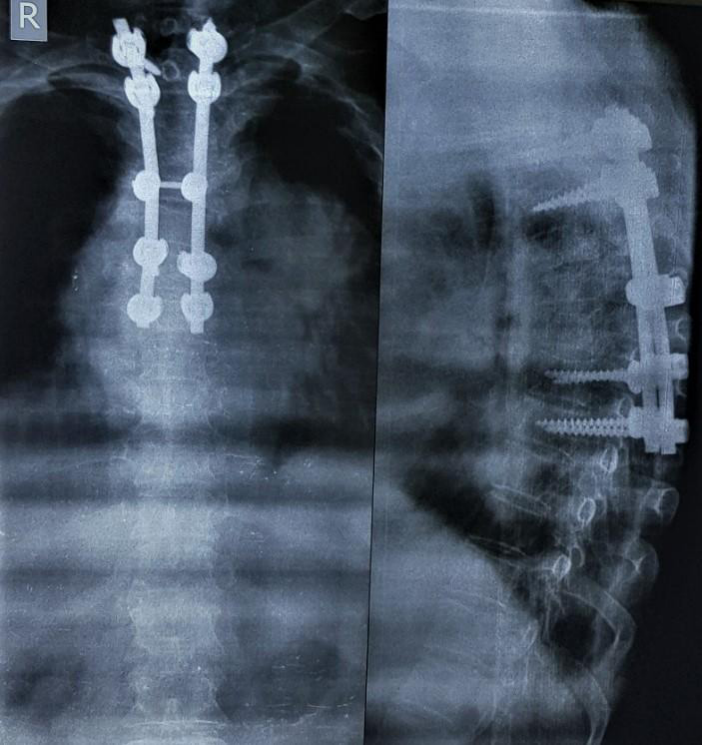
Post-operative Anteroposterior/Lateral x-ray view of dorsal spine showing long segment pedicle screw fixation of the dorsal vertebrae.
